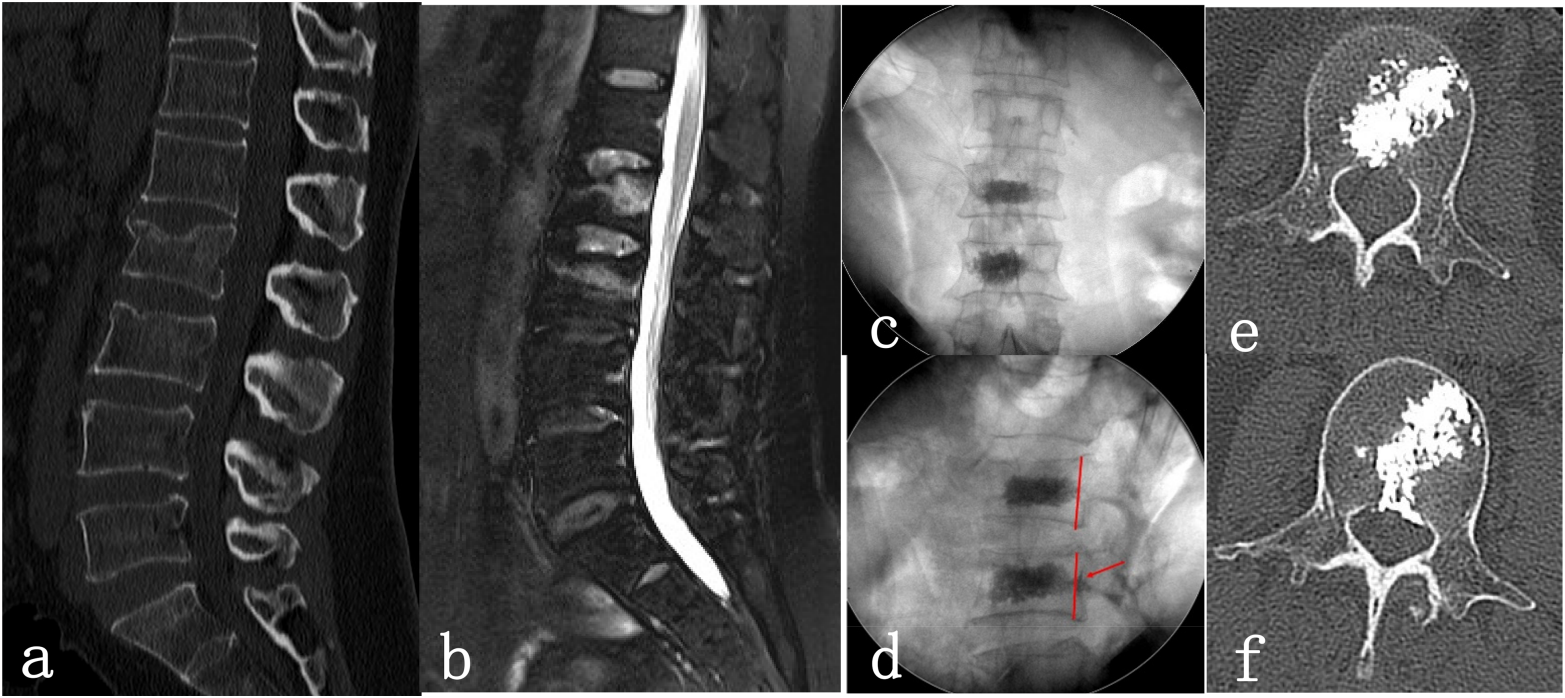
Female patient, 54 years old, suffered from low back pain and limited spinal movement after the fall injury for 2 days. She underwent unilateral percutaneous kyphoplasty (PKP) at L2 and L3. (a,b): Preoperative lumbar CT showed L2 and L3 vertebral fracture lines, and preoperative lumbar MRI showed high signal in the L2 and L3 vertebral bodies; (c,d): Intraoperative x-ray images, lateral fluoroscopy showed L2 bone cement diffusing backwards just enough to reach the warning line (red line), and L3 bone cement diffusing backwards beyond the warning line; (e,f): Postoperative lumbar CT showed that the bone cement in the L2 vertebral body had just spread backwards and reached the posterior wall of the vertebral body without leakage, while the bone cement in the L3 vertebral body showed leakage within the spinal canal.
